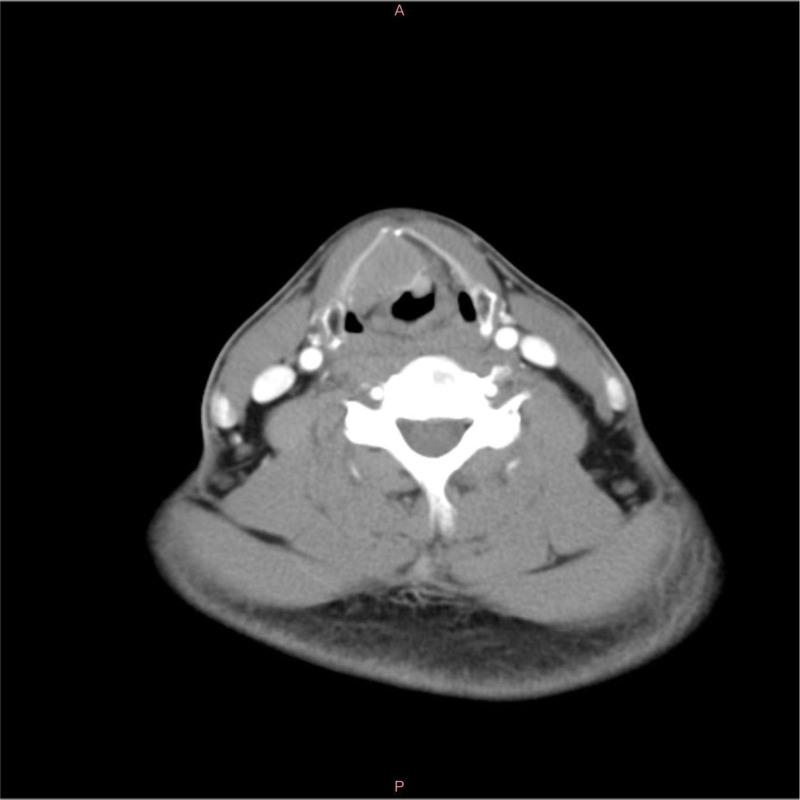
Axial computed tomography (CT) scans of the larynx. This CT scan at the level of the vocal cords displays a well-defined mass in the right paraglottic space. The mass demonstrates homogeneous enhancement and is adjacent to but not invading the thyroid cartilage.
